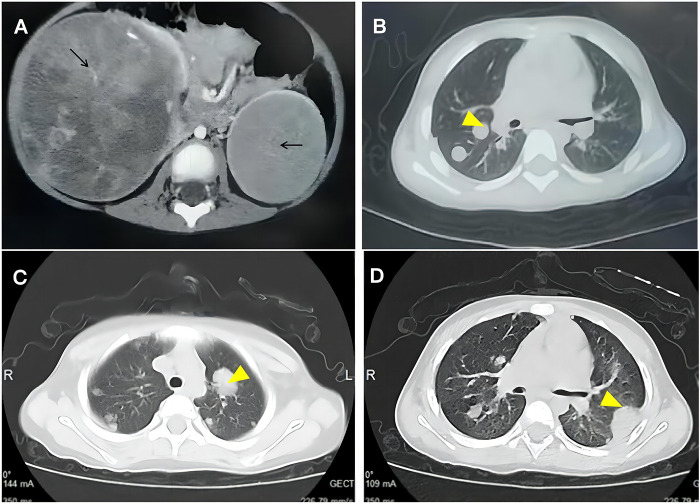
The patient's CT. (A) Right renal mass measuring approximately 9 × 8 × 10 cm, with multiple gravel-like calcifications and focal necrosis areas observed within. Post-contrast imaging shows significant heterogeneous enhancement. The left renal lesion measures approximately 5 × 6 × 6 cm, with focal necrosis observed, and shows uneven enhancement post-contrast; (B) Multiple metastatic tumors in both lungs; (C) Multiple, bilateral, round-shaped nodules were seen in both lungs, with a larger mass observed in the left upper lobe; (D) Multiple nodules and masses were seen in both lungs, with lesions in the right lower lobe and left lower lobe demonstrating irregular margins. Multiple linear opacities were present, suggesting possible interstitial changes.
